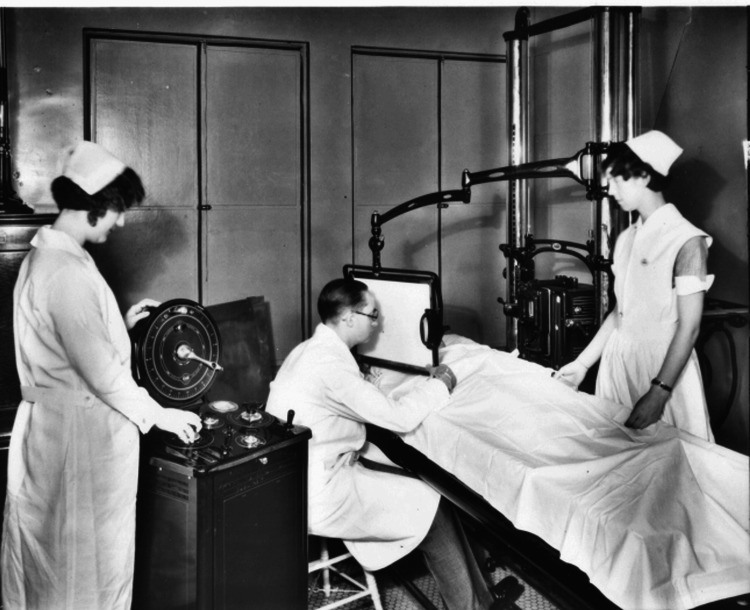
Dr. Alan Hart is depicted operating an X-ray machine at Tacoma General Hospital in the early 1930s, highlighting his contributions to medical imaging. Used with permission from Tacoma Public Library’s Northwest Room, Richards Studio Photographs (Collection 2.1.1), item 647-9. https://tpl.accesstomemory.org/647-9.
